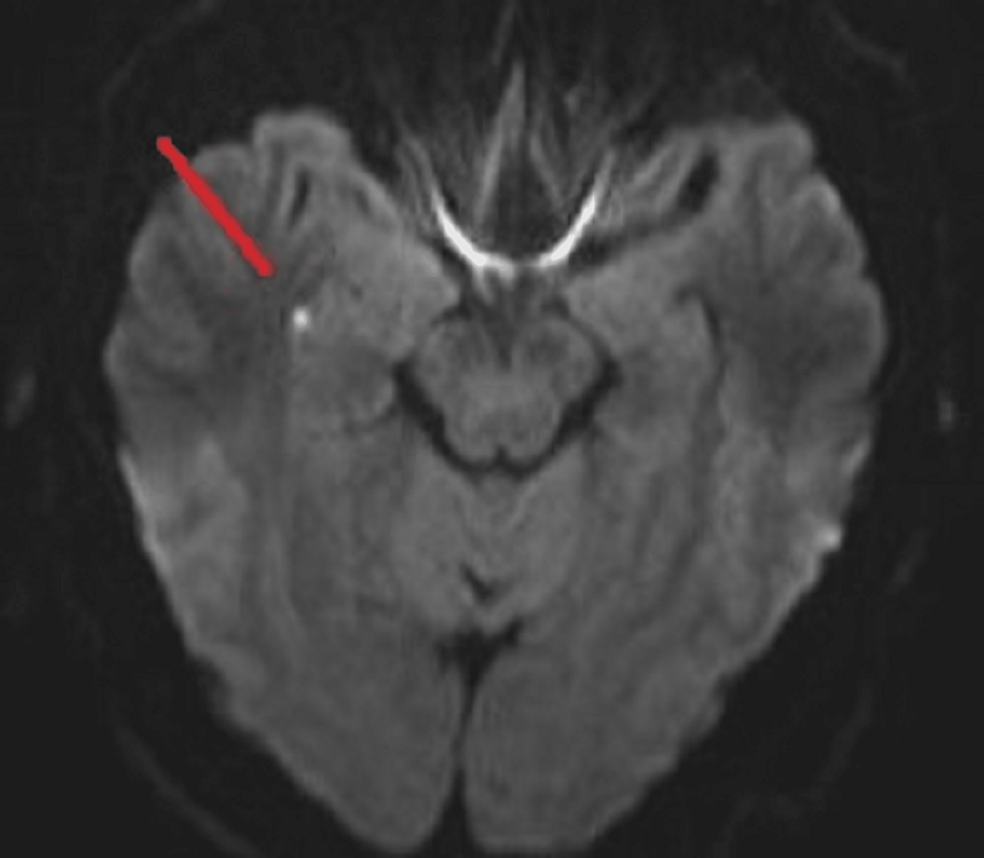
Magnetic resonance diffusion-weighted imaging (DWI) showing a punctate area of diffusion restriction in the medial right temporal lobe 13 hours after the episode
